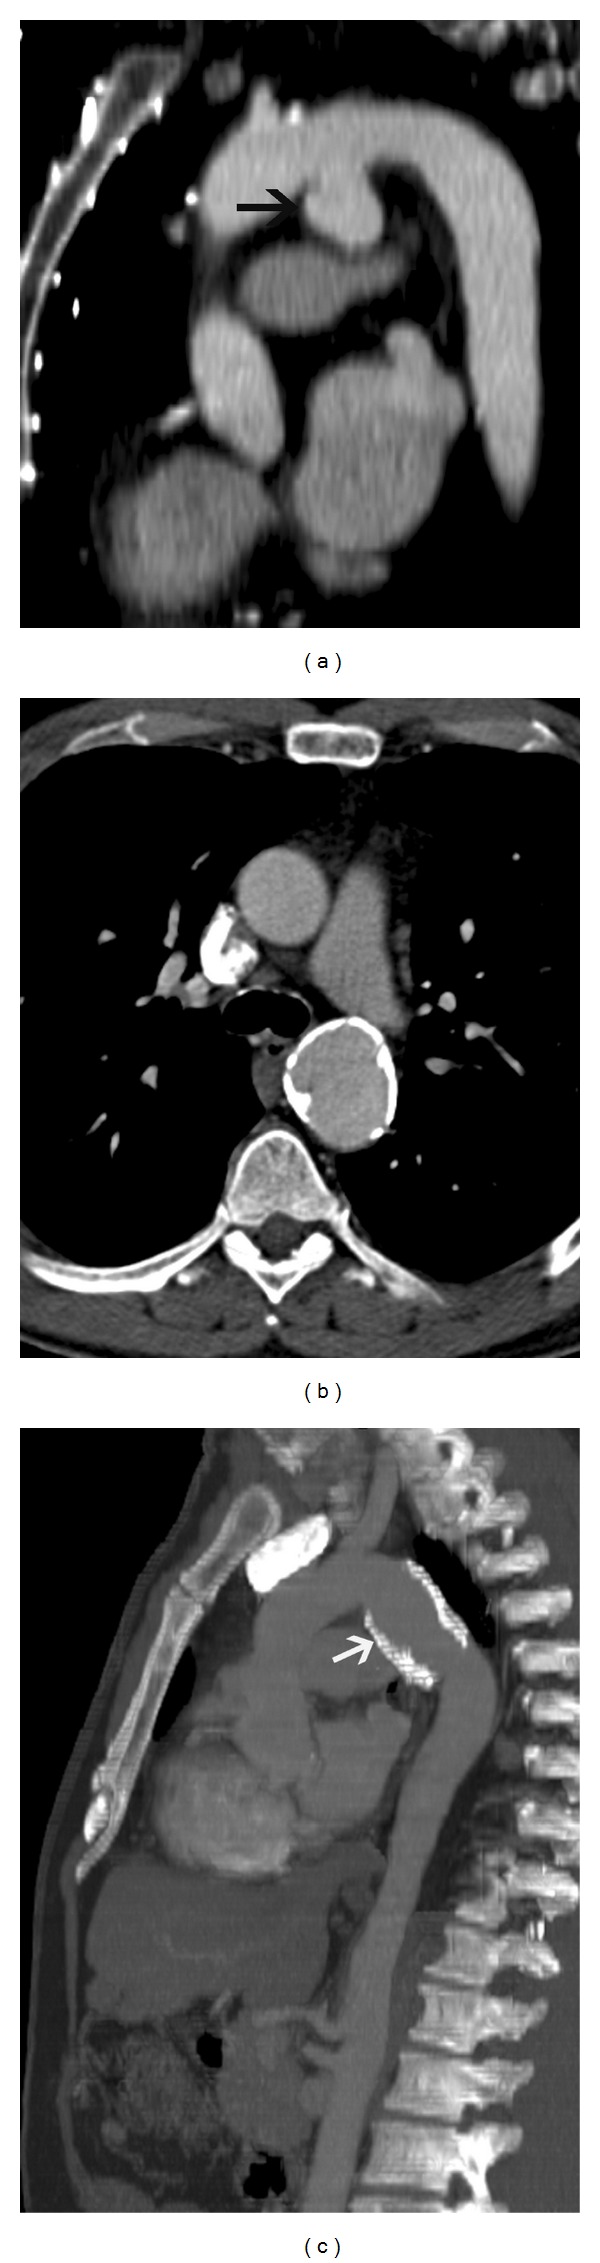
Chronic traumatic aneurysm—(a) sagittal reconstructed CT image in a 71-year-old male involved in road traffic accident five years back shows a saccular pseudoaneurysm in the aortic isthmus. (b) Axial CT image in a 65-year-old male involved in motor vehicle accident ten years back shows a calcified aneurysm of the aortic isthmus. (c) Sagittal reconstructed CT image in a 65-year-old male involved in motor vehicle accident ten years back shows a calcified saccular pseudoaneurysm in the aortic isthmus.
